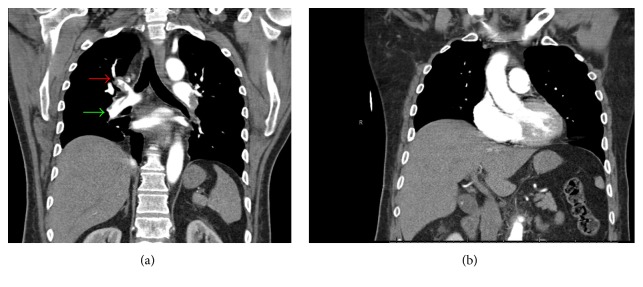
Perioperative chest computed tomography angiography; coronal reconstructions. (a) Filling defects were noted in right upper (red arrow) and lower lobar (green arrow) pulmonary arteries. (b) Right ventricular dilatation.
